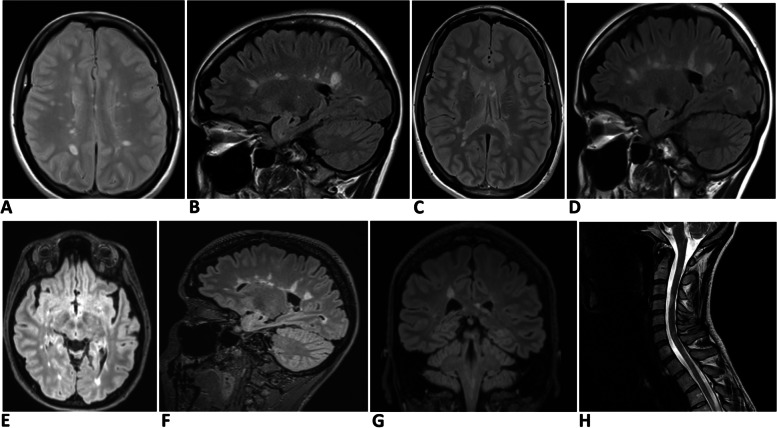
Serial MRI changes of case 2. A & B Brain scan (axial T2 and sagittal FLAIR sequences respectively) at the time of the diagnosis of MS. Note multiple pericallosal lesions consistent with Dawson’s fingers. C & D Brain scan (axial T2 and sagittal FLAIR sequences respectively) six years later when Alemtuzumab therapy was commenced. Note changes similar to A & B. E–G Images when MOG antibody test was found to be positive. E (brain axial FLAIR), F (sagittal FLAIR), and F (coronal FLAIR) shows multiple pericallosal lesions. H (sagittal T2 spine) shows multiple lesions at cervico-medullary junction, C2, C4, C6, and upper thoracic level
